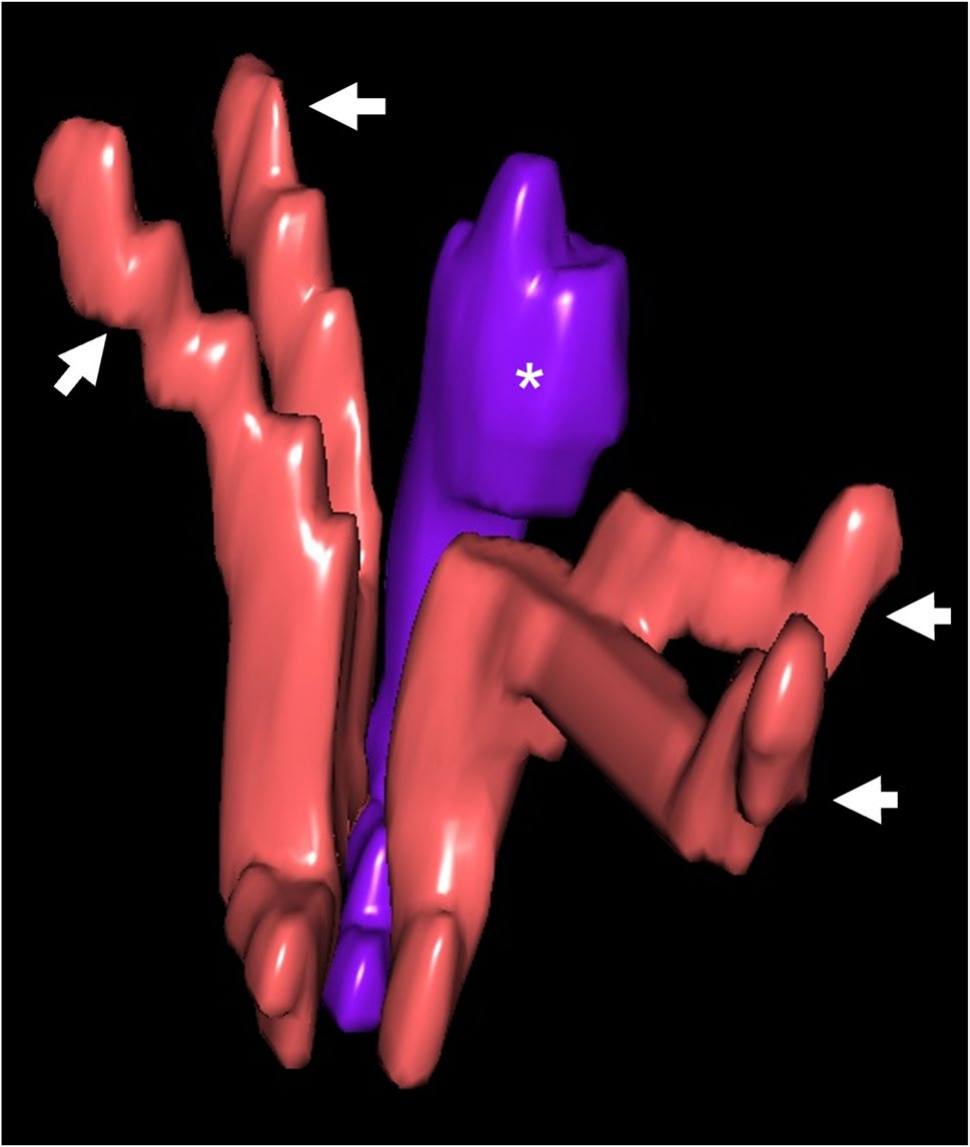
4-month-old ischiopagus conjoined twin boys with a common penis (same patients as in Figs. 7 and 8). Magnified 3-diemsnional view of the corporeal tissue extracted from axial high resolution T2-weighted magnetic resonance imaging. There are four corpora cavernosa (two belonging to each twin) *(arrows*) and a common corpus spongiosum (*asterisk*). (Visible Patient®, Strasbourg, France)
